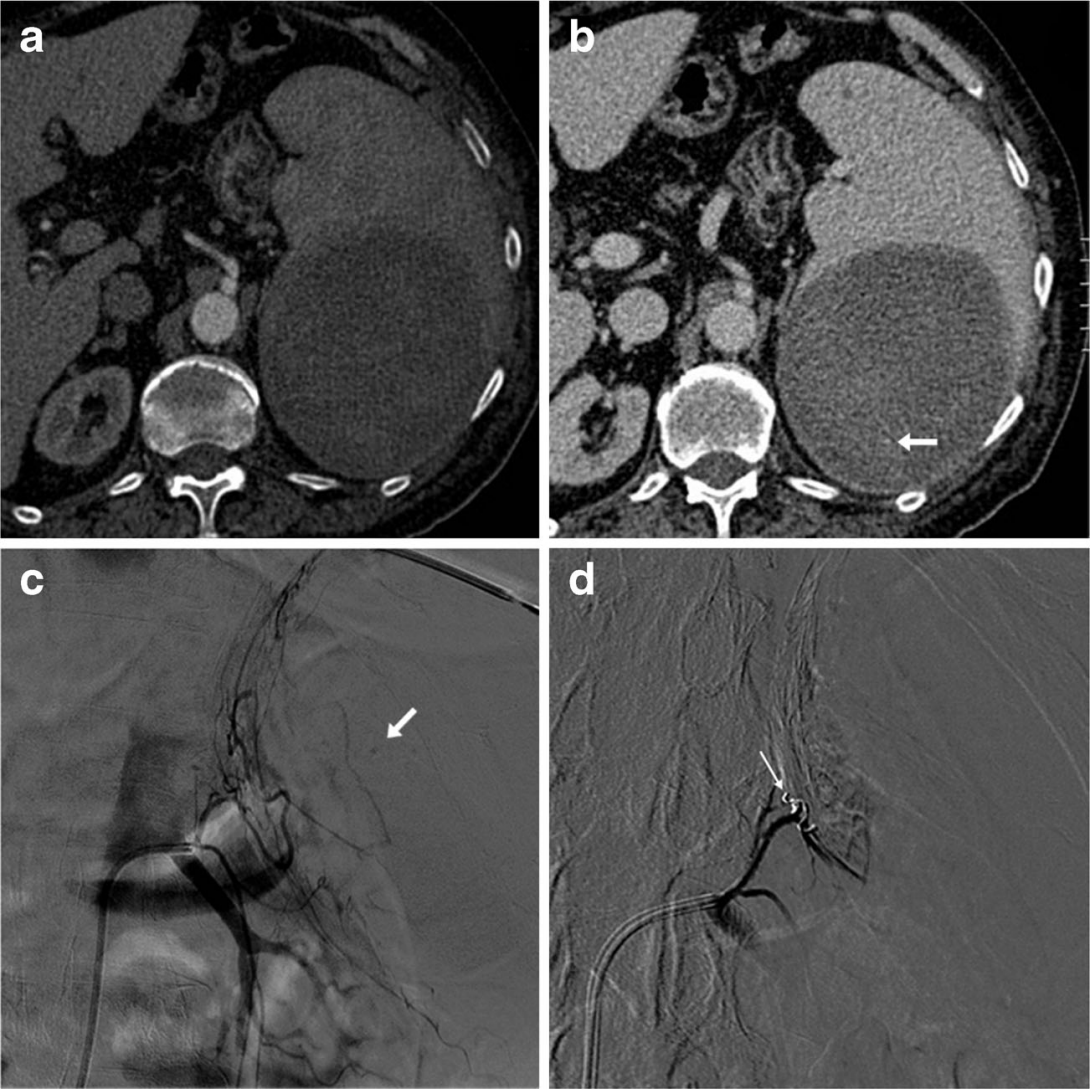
A 73-year-old male on anticoagulation suffered from spontaneous acute abdominal pain. His medical history included congestive heart failure, complete atrioventricular block treated with an implantable cardioverter-defibrillator, previous angioplasty and coronary stenting for acute myocardial infarction, and surgically treated colon carcinoma. Physical examination revealed hypotension and tender left hypocondrium. The international normalized ratio (INR) was 2.6; haemoglobin level was 6.5 g/dl. CT (a, b) revealed a large, roundish mixed attenuation intraparenchymal splenic haematoma without haemoperitoneum. Active bleeding was not evident during the arterial-phase acquisition (a). Faint contrast “blush” (arrow in b) was visible in the venous phase and confirmed at urgent arteriography as minimal bleeding originating from the left phrenic artery (arrow in c). Selective embolization with Spongostan and coil (thin arrow in d) was performed and allowed successful nonoperative management
